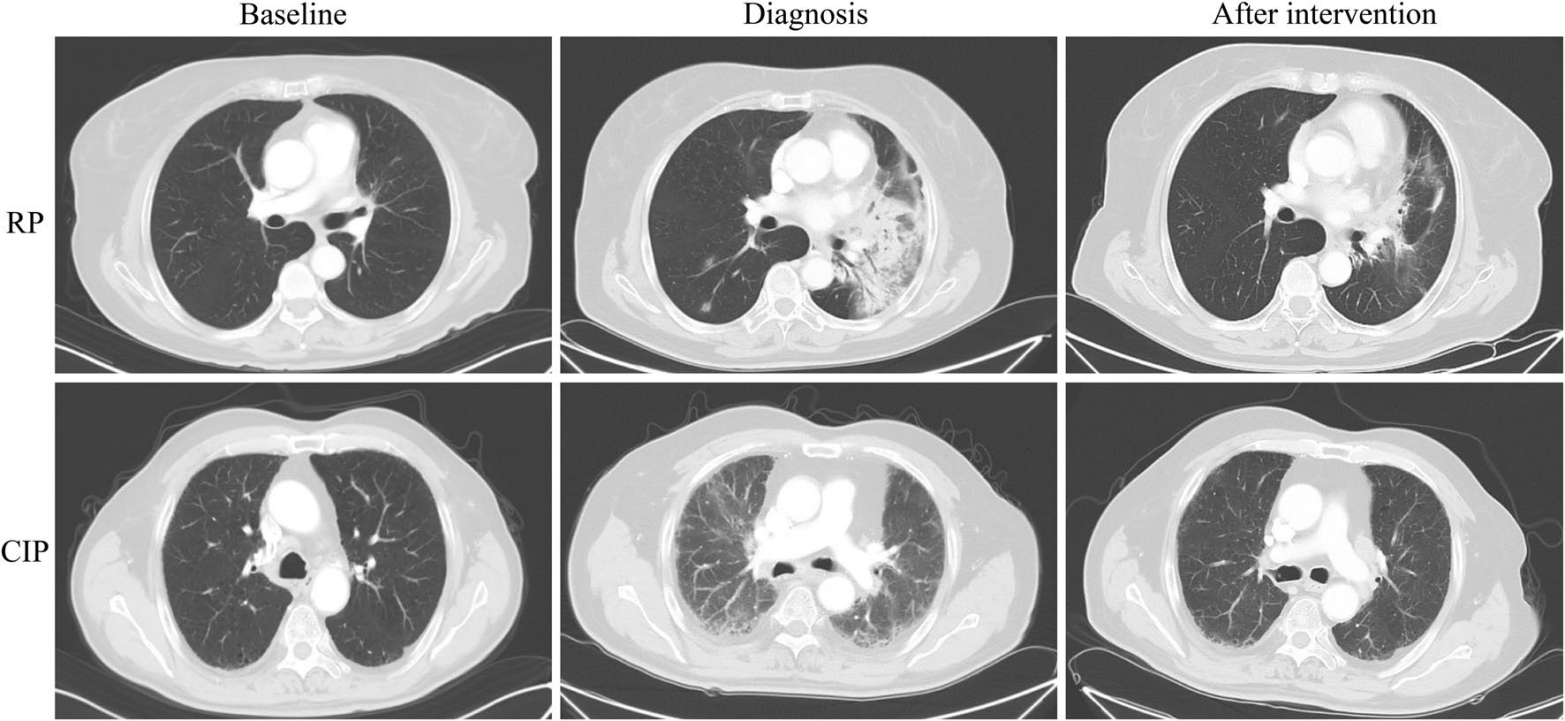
Representative CT changes of patients with RP and CIP. RP: radiation pneumonitis; CIP: checkpoint inhibitor pneumonitis
